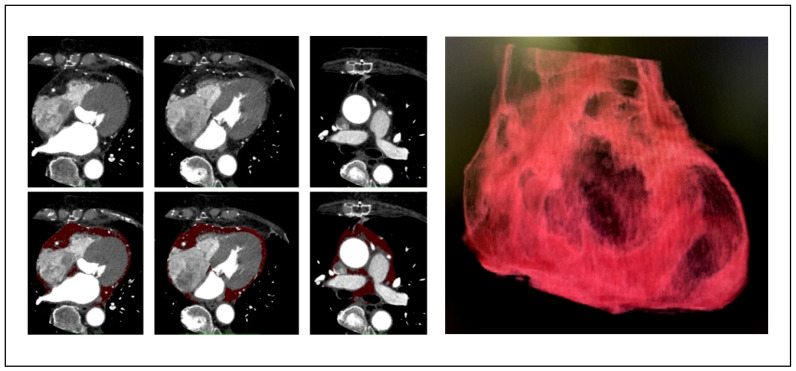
Definition of EAT on CT. EAT was defined as adipose tissue between the myocardium and pericardium. Representative axial CT images show EAT (highlighted area) with attenuation values of −195 to −15 Hounsfield units. EAT, epicardial adipose tissue; CT, computed tomography.
